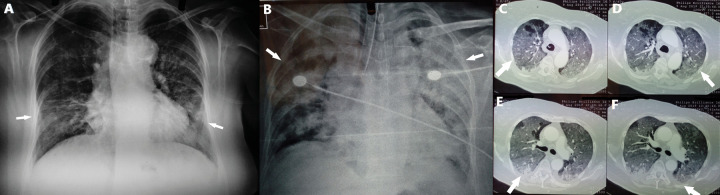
A: Chest X-ray shows patchy diffuse infiltrates in both lungs mostly in the left lower lobe on admission. B: *Chest X-ray shows diffuse lung infiltrates after two days of hospitalization.* *C, D: *Chest Computed Tomography reveals diffuse ground glass opacities. E, F: Chest Computed Tomography reveals pleural effusion in both lungs.
